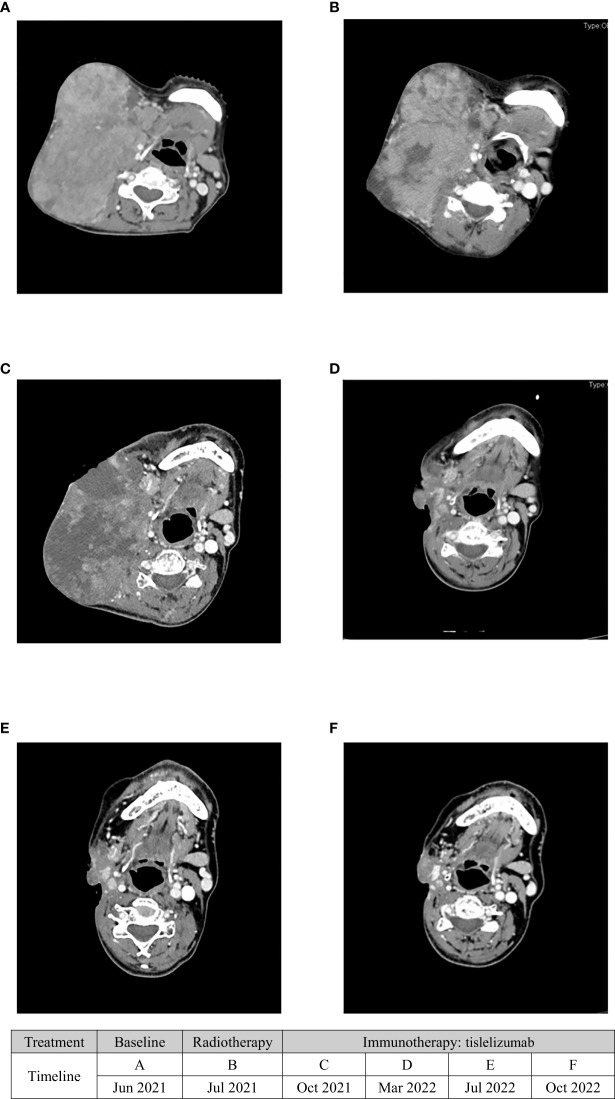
The serial CT images of the patient before and after treatment with radiotherapy and tislelizumab immunotherapy. (A) CT image of right neck lymph node recurrence in June 2021(baseline). (B) CT image of the right neck lymph node in July 2021 after radiotherapy. (C) CT image of the right neck lymph node in October after tislelizumab immunotherapy. (D) CT image of the right neck lymph node in March 2022. It showed the tumor shrank. (E) CT image of the right neck lymph node in July 2022. (F) CT image of the right neck lymph node in October 2022.
